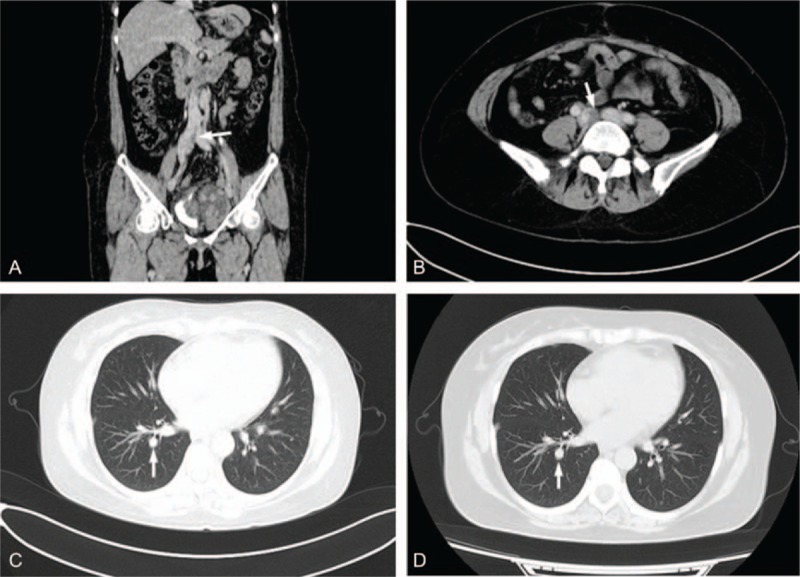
Computed tomography images of case 2 were shown. A pelvic mass and filling defect right common iliac vein and inferior vena cava (A and B) were detected in March 2014. Multiple round-shaped nodules were randomly distributed in bilateral lungs in March 2014 (C). Multiple nodules in bilateral lungs remained stable in April 2015.
